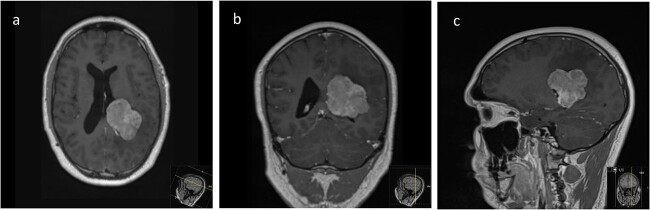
Pre-operative axial (a), coronal (b), and sagittal (c) T1-weighted post contrast MRI sequences demonstrating the avidly enhancing lesion in the posterior horn of the left lateral ventricle, with extension into the periventricular white matter.
